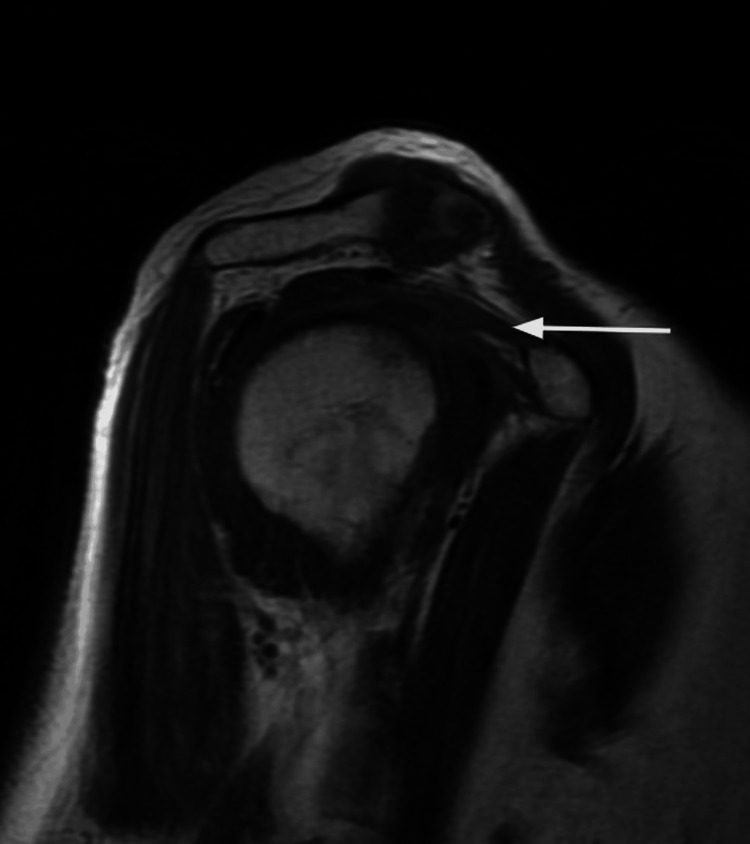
Sagittal T1-weighted MRI. The white arrow indicates thickening of the coracohumeral ligament.
